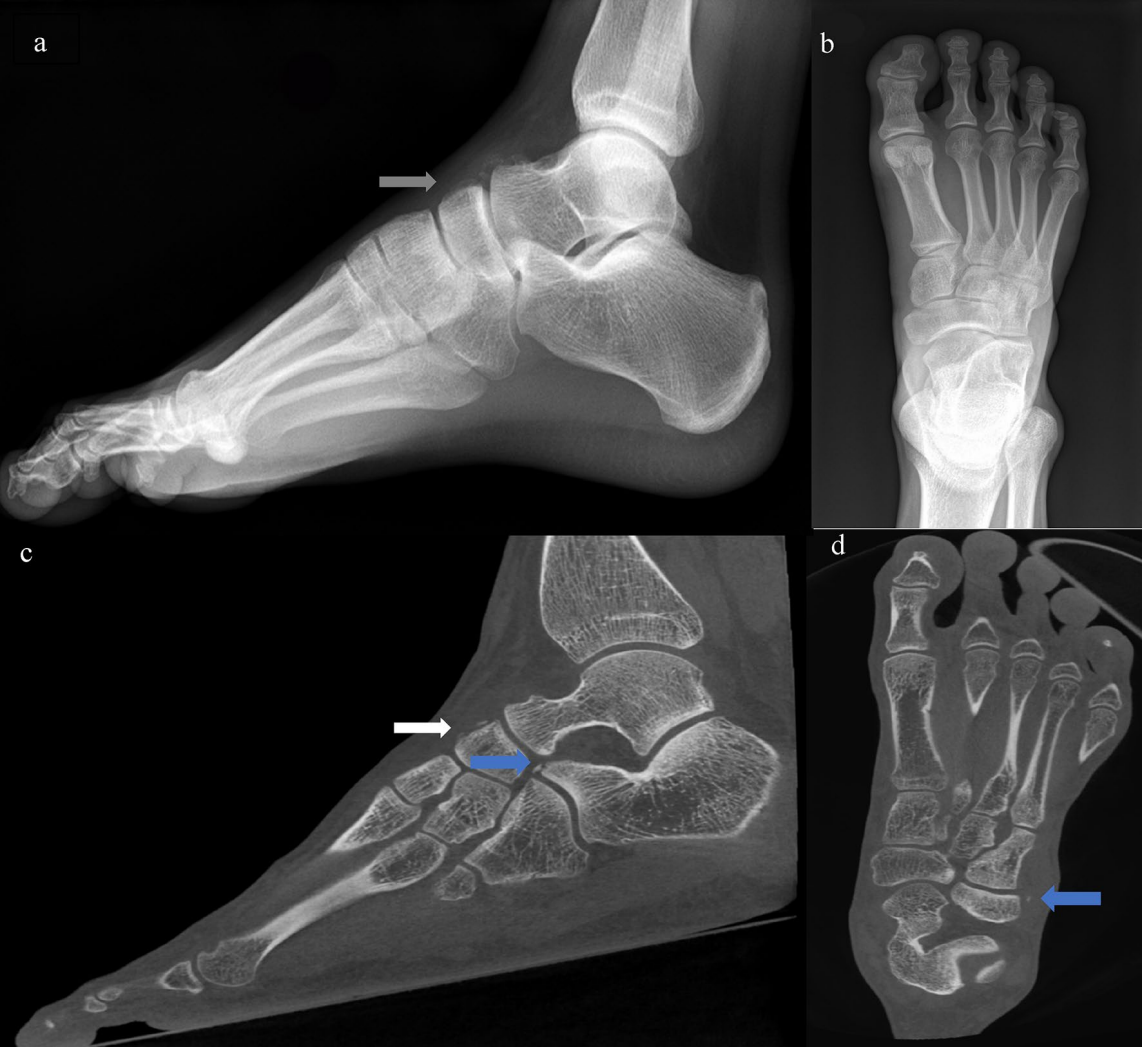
Standard XR a sagittal and b axial in a patient with multiple Chopart involvement not visible on the XR and found on CBCT’s multiplanar reformation (MPR) c sagittal and d axial. The gray arrow shows a talonavicular fracture/avulsion visible on XR. The white arrow points to a talonavicular fracture/avulsion visible on XR and CBCT. The blue arrow shows a fracture/avulsion of the anterior calcaneal process and calcaneocuboid ligament avulsion not visible on XR
